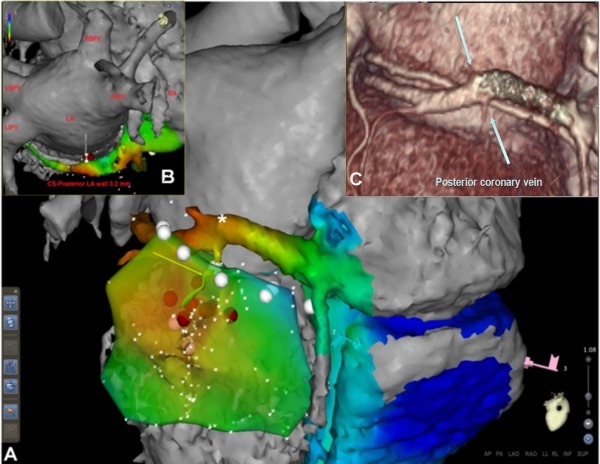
A.- Left ventricle (LV) epicardium and coronary sinus (CS) electroanatomical map merged with computed tomography scan. Red dots represent ablation attempts at the accessory pathway's epicardial ventricular insertion. The yellow arrow highlights the course of a posterior coronary vein from the successful ablation site (*) to the epicardial ventricular insertion site. B.- The successful ablation point inside CS from a cranial-posterior view is shown. C.- 3D reconstruction of CS anatomy obtained from CT scan. (*) indicates the successful ablation site. Light blue arrow highlights a posterior branch of the CS. CS: coronary sinus; LA: left atrium; LSPV: left superior pulmonary vein; LIPV: left inferior pulmonary vein; RSPV: right superior pulmonary vein; RIPV: right inferior pulmonary vein; RA: right atrium.
